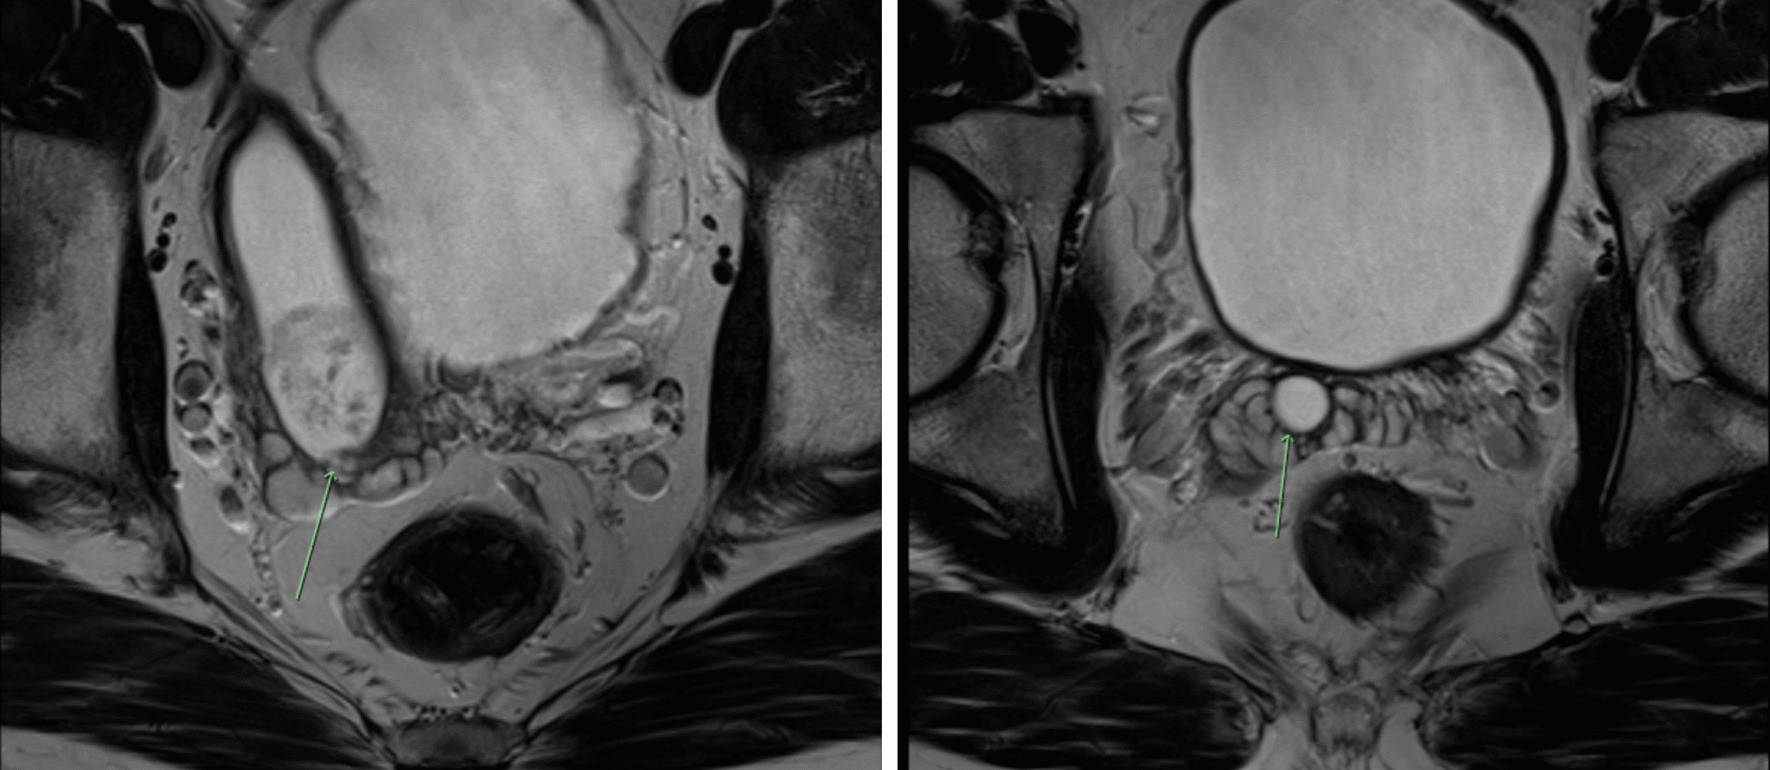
MRI images demonstrating cystic papillary adenoma of Seminal Vesicle
